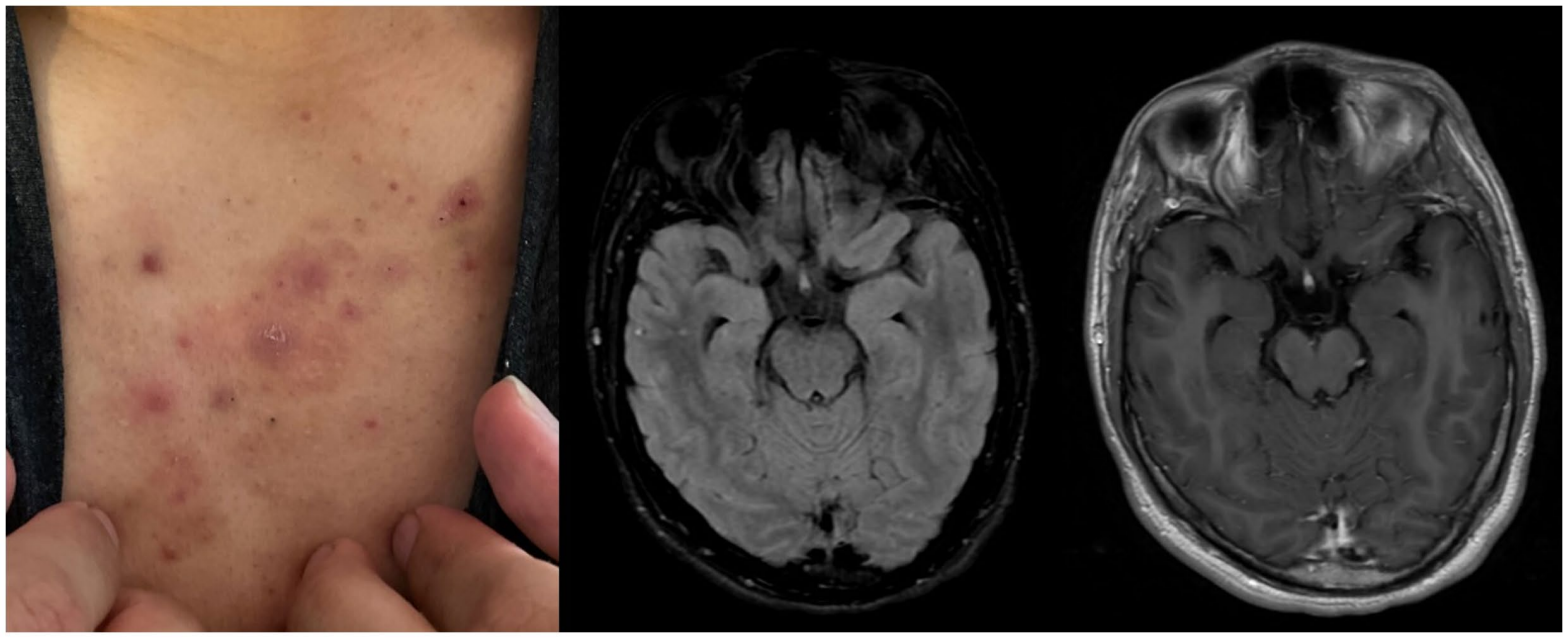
(A) Maculopapular rash (chest). (B) Ax FLAIR (T2) pre contrast, no abnormal signal enhancement. (C) Post contrast AxT1 FLAIR, no abnormal contrast enhancement.
